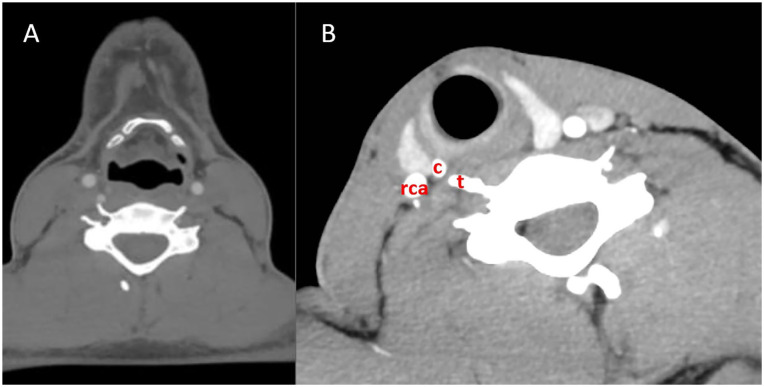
(A) Computed tomography (CT)-scan of the neck in neutral position, axial
